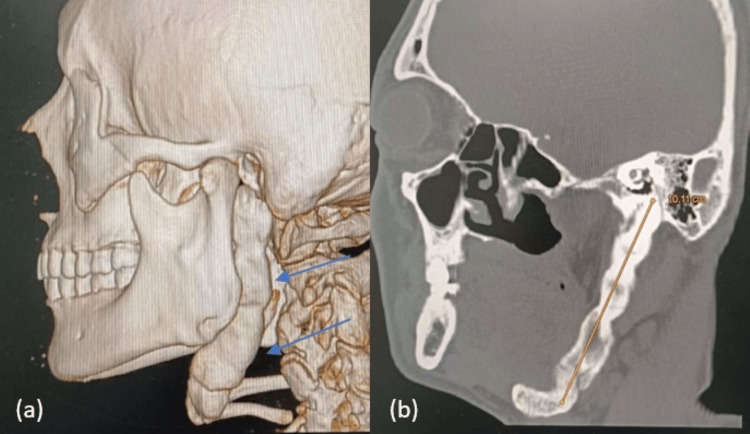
(a) 3D- reconstructed view of the left styloid process; (b) Sagittal cone-beam computed tomography (CBCT) slice showing measurement of the left styloid–stylohyoid complex (10.11 cm)
